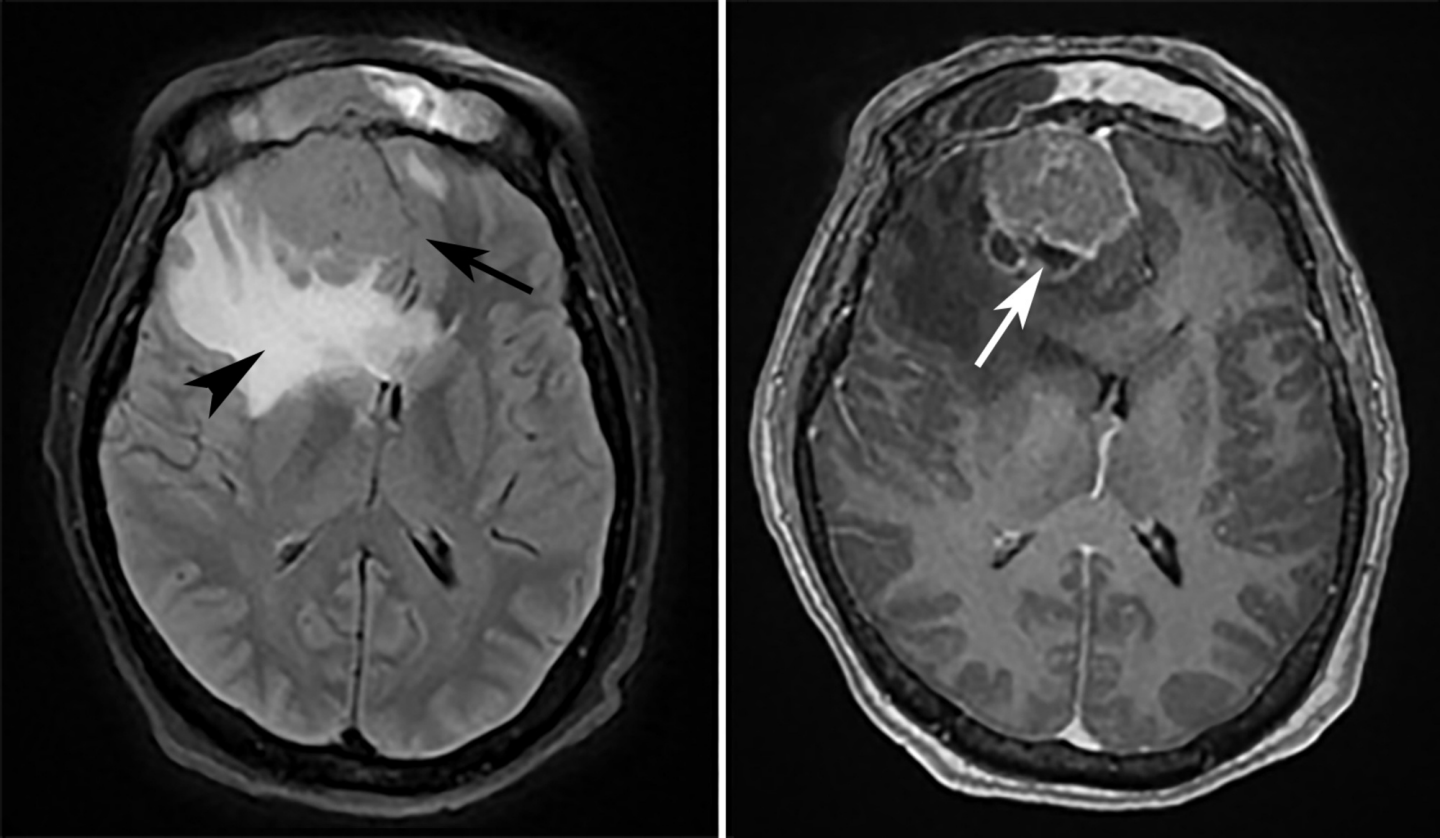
MRI head, T2 FLAIR, and T1 post-contrast Axial T2-weighted FLAIR (*left*) and T1-weighted post-contrast (*right*) MRI images demonstrate a soft tissue mass within the right frontal lobe with mass effect on the medial left frontal lobe (*black arrow*). T2 FLAIR hyperintense signal surrounding the mass (*arrowhead*) is consistent with vasogenic edema. T1 rim and heterogeneous central enhancement is visualized, with an area of hypointense signal at the posterior margin, suggestive of necrosis (*white arrow*). FLAIR: fluid-attenuated inversion recovery, MRI: magnetic resonance imaging
